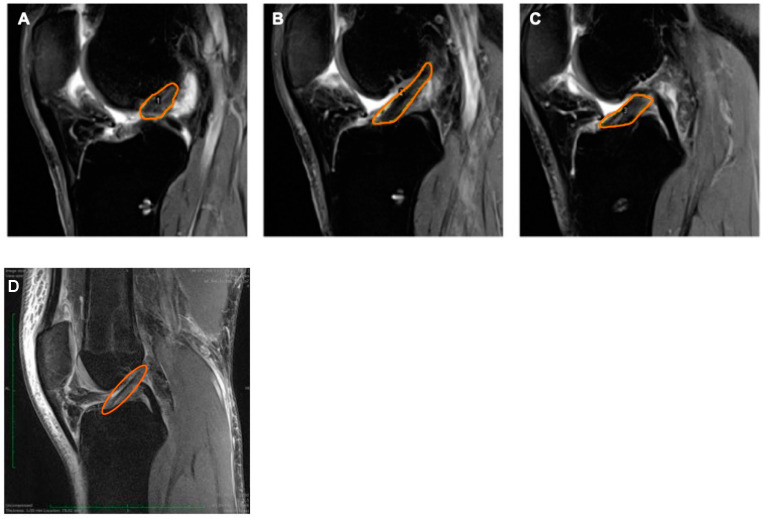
Sagittal MRI scans (T2 weighted sequence) with marked (orange) ACL as region of interest for signal intensity ratio calculations. (A) SIR lateral to the PCL, (B) SIR at level of PCL, (C) SIR medial to the PCL, and (D) intact (healthy) ACL.
